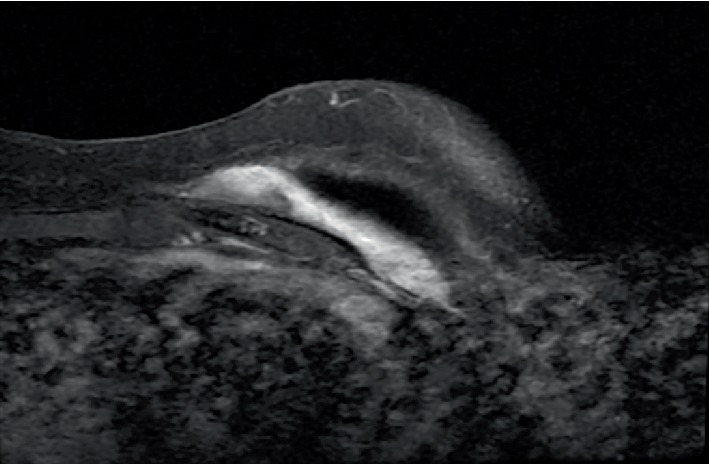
T1-weighted post-contrast MRI of the left breast demonstrates a well demarcated soft tissue mass posterior to the silicone breast implant.
